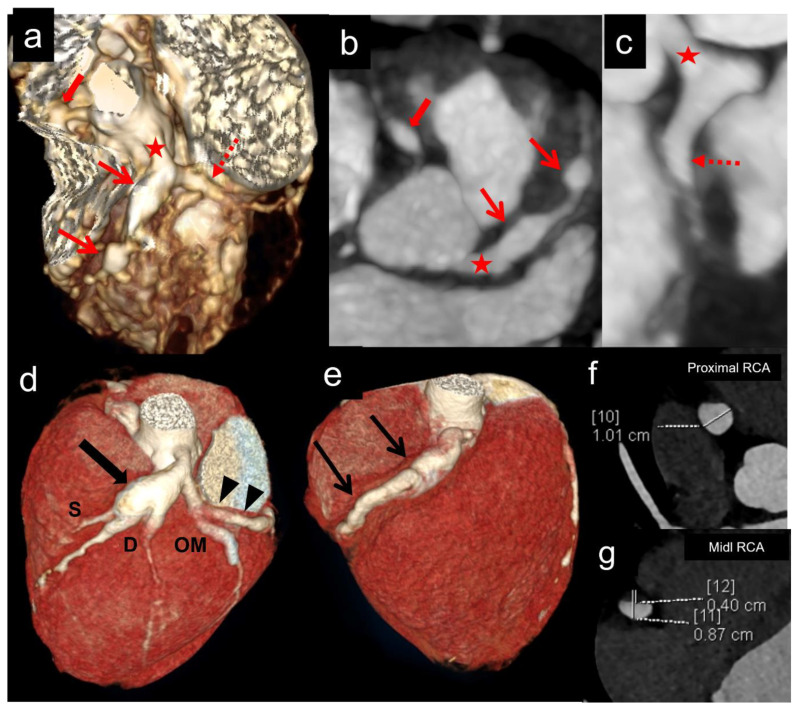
(a–c): (Patient No.12 in Table 2): CT coronary angiography (CTCA) volume rendered (a), axial (b) and curved reformatted images of left circumflex (LCX) (c) in a 2 months female infant at presentation show fusiform aneurysm of left main coronary artery (LMCA) (asterisk in a–c) with extension into osteo-proximal segments of LAD (thin arrow in a,b) and LCX (interrupted arrow in a,c). Another non-contiguous aneurysm is seen in mid LAD (second thin arrow in a,b). Although TTE showed aneurysms in LMCA, proximal LAD, and RCA, it failed to demonstrate a second non-contiguous aneurysm in mid LAD and a fusiform aneurysm in LCX. (d–g): (Patient No.22 in Table 2): CTCA volume rendered (d,e), axial proximal RCA (f), and distal RCA (g) images in a 96 months boy at presentation show fusiform aneurysm of LMCA with extension into osteo-proximal segments of LAD (thick arrow in d) and LCX (arrow heads in d). Note the extension/involvement of septal (S) and diagonal (D) branches of LAD and the obtuse marginal branch (OM) of LCX (a). RCA shows a fusiform aneurysm in its entire course (thin arrows in e). Axial images of RCA (f,g) show dilated RCA with eccentric thrombus in mid RCA (g). TTE showed aneurysms in LMCA, LAD, and RCA; however, it failed to demonstrate LCX aneurysm, distal extension of RCA aneurysm, and extension/involvement of septal (S) and diagonal (D) branches of LAD and obtuse marginal branch (OM) of LCX. The thrombus in the mid RCA aneurysm was also missed.
